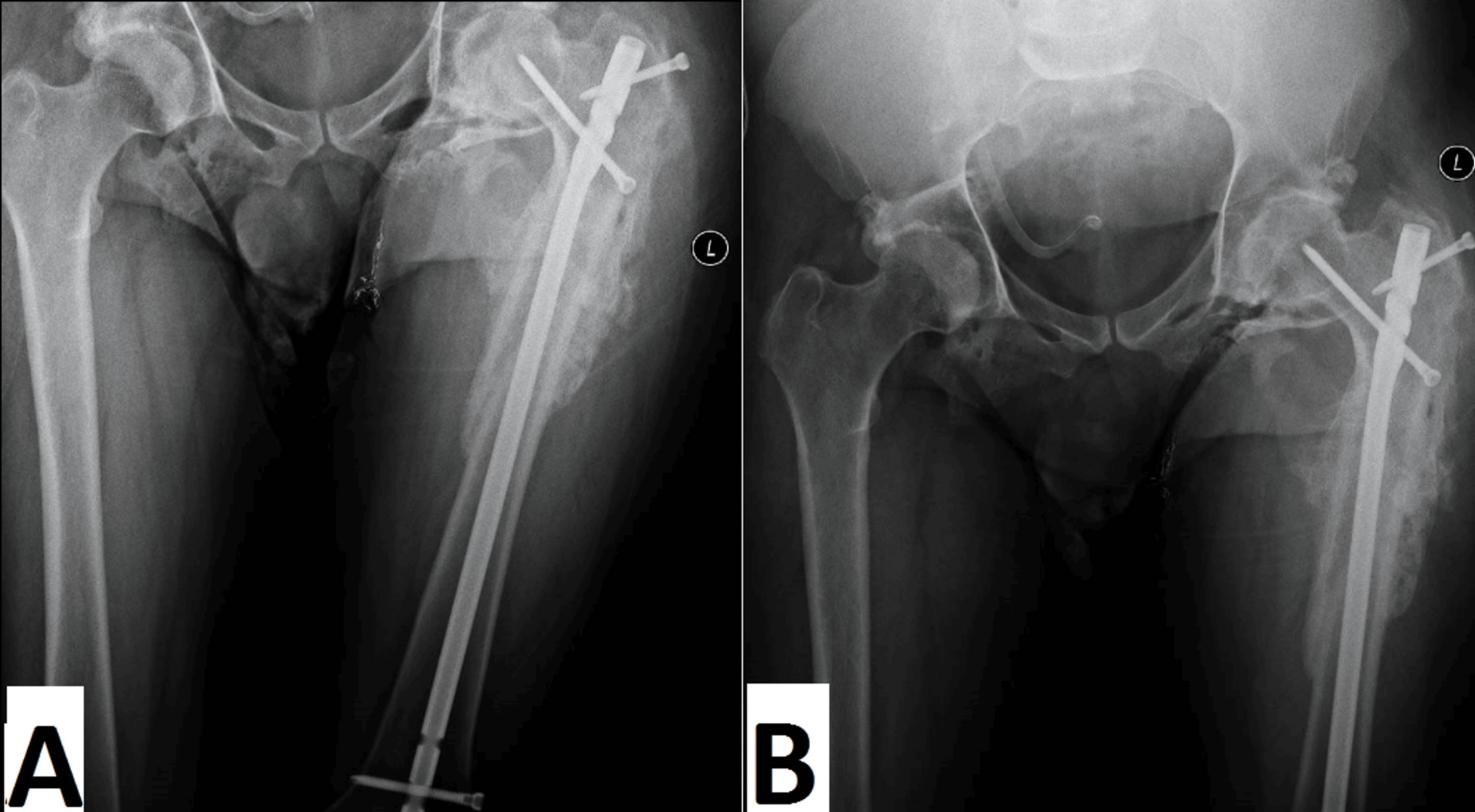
Follow-up plain X-ray, four months postoperative, confirms complete fracture healing.
